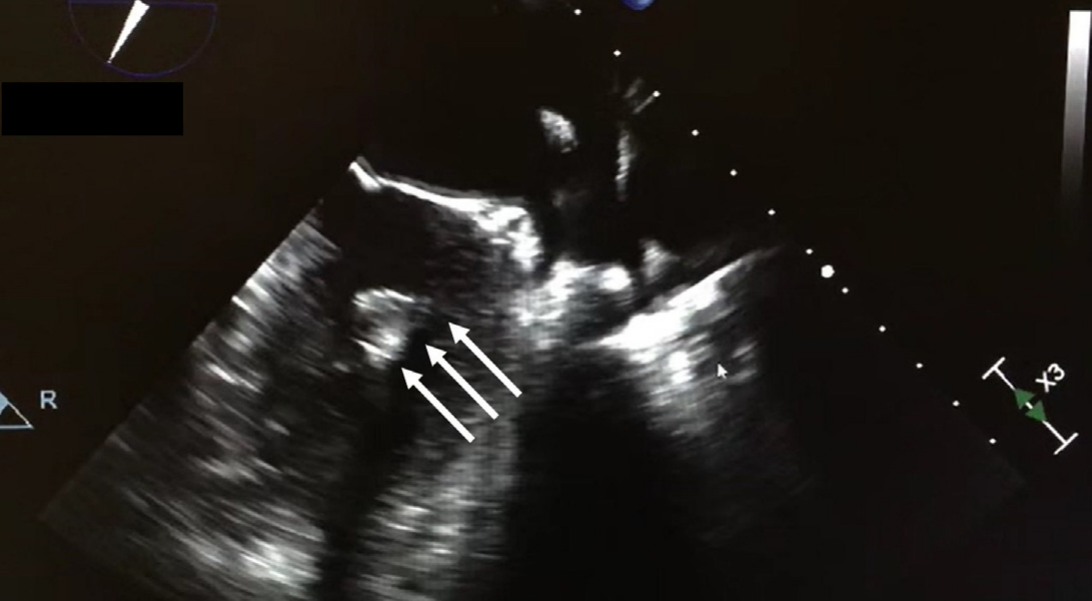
Preoperative transesophageal echocardiogram showing the left ventricular mass on the inferolateral wall (arrows).
